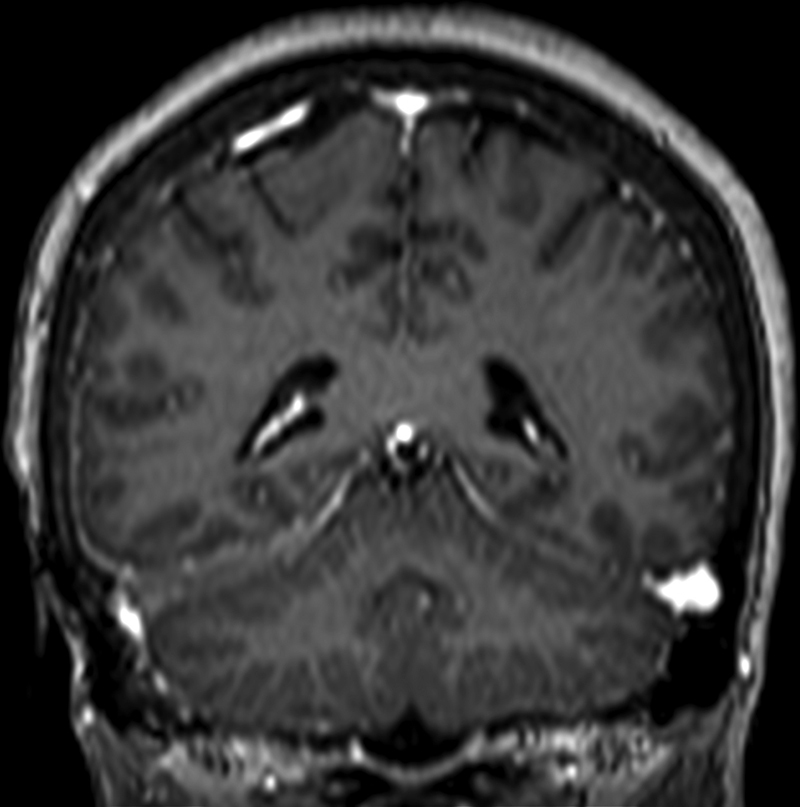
Postcontrast weighted MRI scan showing improvement in dural thickening at the right side on coronal view after treatment with rituximab.
